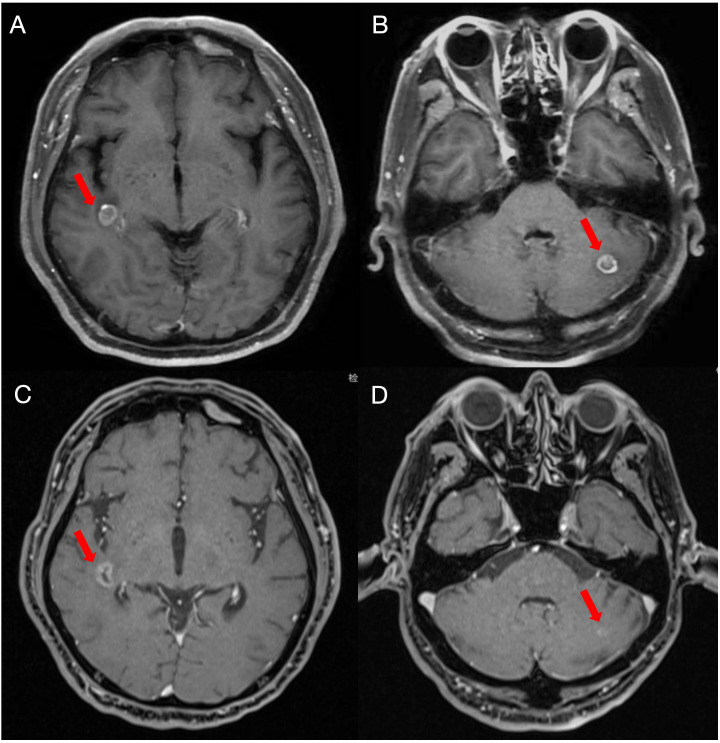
Right temporal lobe metastasis (A) and left cerebellum metastasis (B) on MRI before radiotherapy. Right temporal lobe metastasis (C) and left cerebellum metastasis (D) on MRI after radiotherapy.
